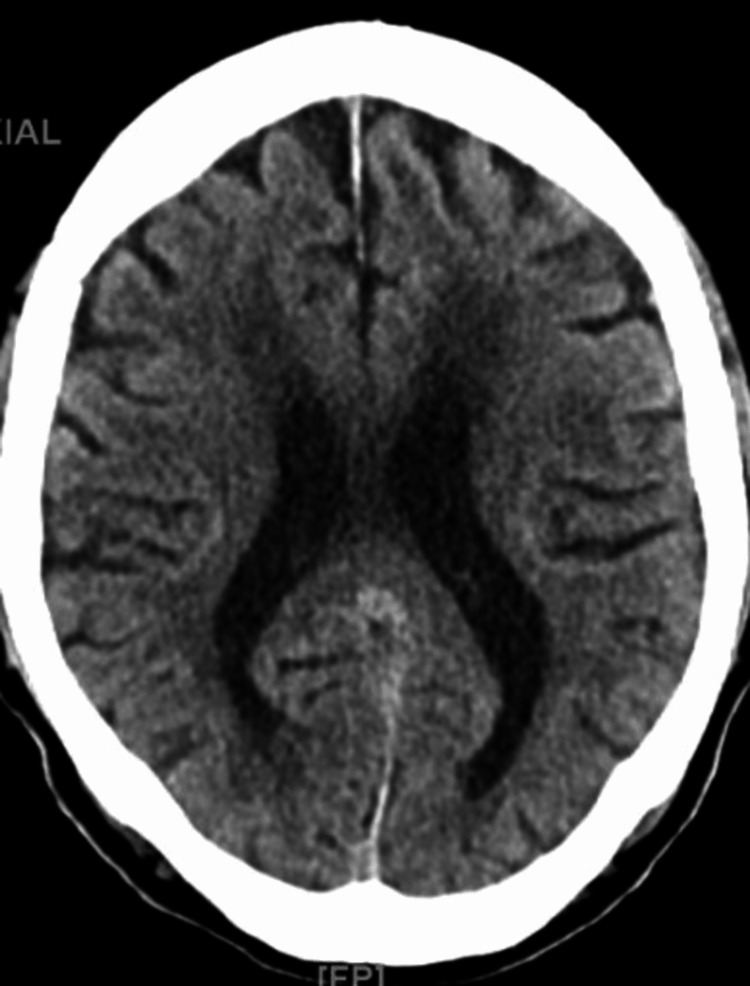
CT head showing bilateral white matter hypodensities compatible with chronic ischemic/degenerative changes, cerebral and cerebellar volume loss, and no acute stroke or evidence of hemorrhage, chronic lacunar infarct in right periventricular white matter is noted measuring up to 6 mm.
